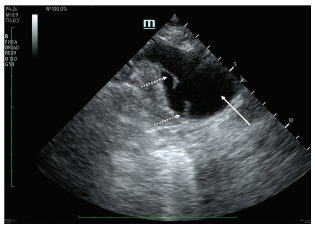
Suprasternal ultrasound visualizing the aortic arch in transection (white arrow) with a non-contiguous dissection flap at the 8 and 10 o’clock positions (dashed arrow) representing the aortic mural defect causing dissection.
